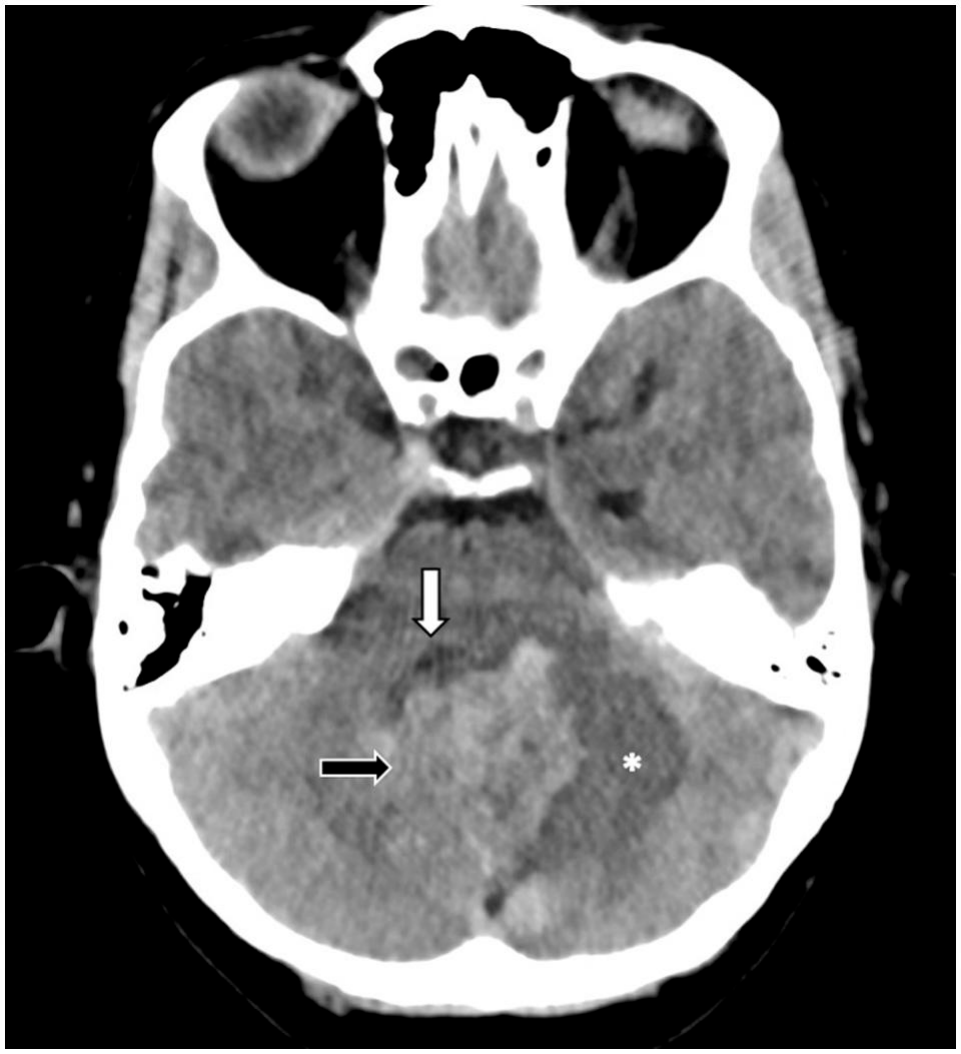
Non-contrast head CT shows a heterogeneous and hyperdense multilobulated mass (black arrow) in the left cerebellar hemisphere, with perilesional edema (asterisk) and compression on the fourth ventricle (white black).
